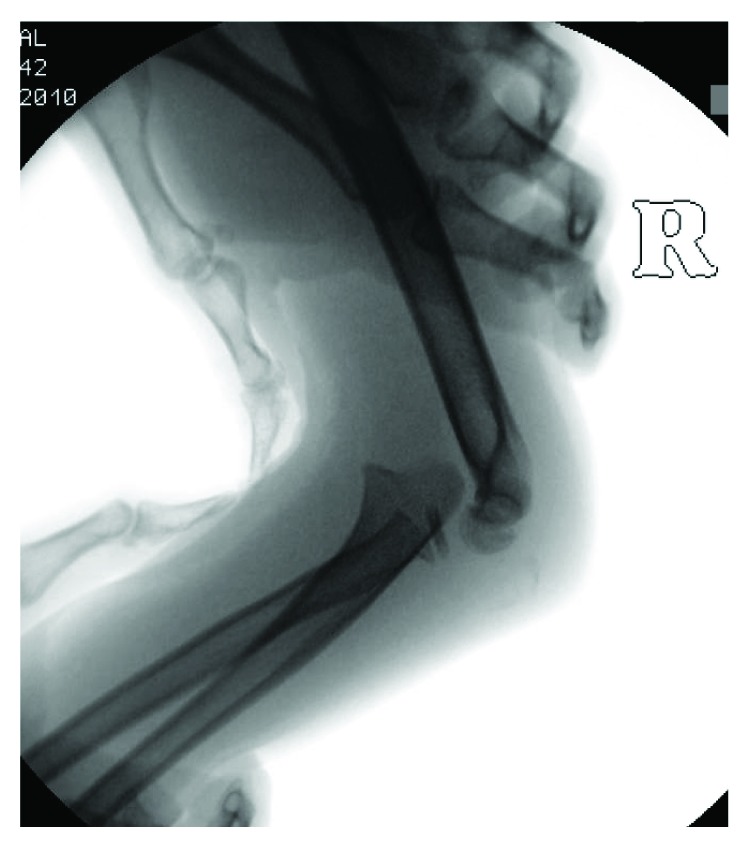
Lateral radiograph of the right elbow showing continued anterior dislocation of the ulnohumeral joint despite attempted reduction. The Salter-Harris II fracture of the radial head can again be noted.
